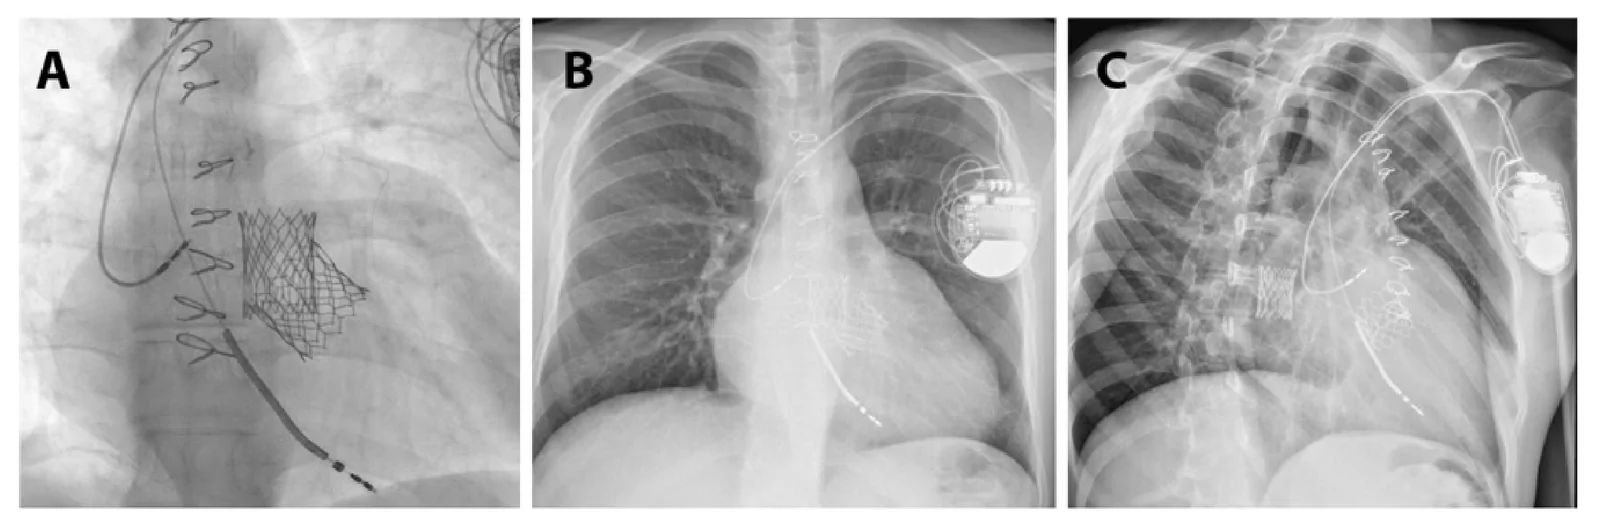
Imaging after transcatheter aortic valve implantation. (A) Final fluoroscopic image demonstrating successful implantation of the second Edwards SAPIEN 3 valve in the native aortic annulus after embolization of the first prosthesis to the descending thoracic aorta. (B) Frontal chest radiograph and (C) 60° right anterior oblique chest radiograph confirming stable valve position at follow-up.
